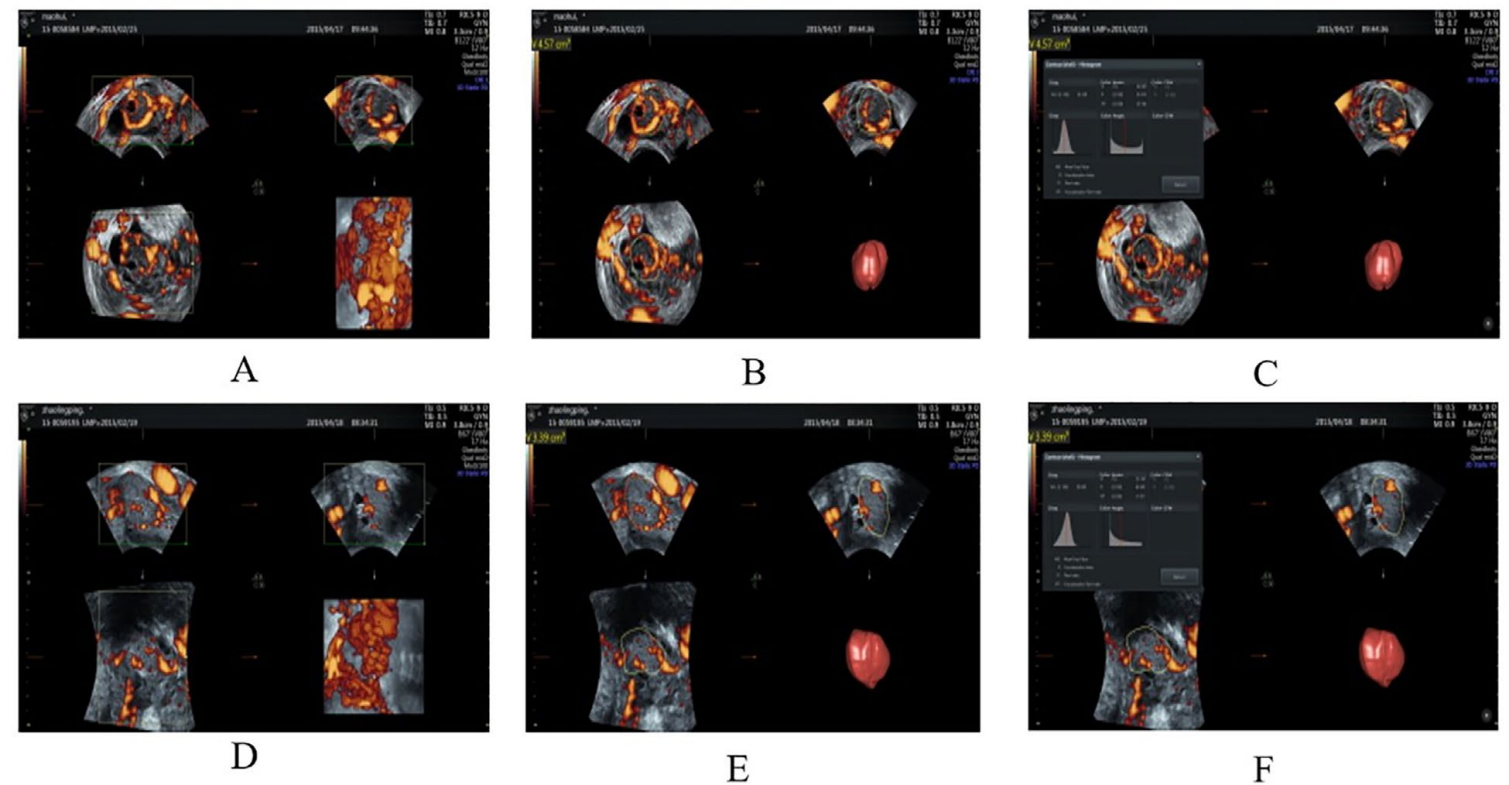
Three-dimensional ultrasound images of corpus luteum in group A and group B. (A, B) The 3D-PD ultrasound images of the corpus luteum in group A (27 y, G1P0, menolipsis 69 days, no symptoms of abdominal pain or vaginal bleeding, intrauterine pregnancy, having yolk sac, germ, and fetal heart beating). (C) The histogram of gestational corpus luteum in the same patient. (D, E) The 3D-PD ultrasound images of the corpus luteum in group B (34 y, G1P0, menolipsis 59 days, with the symptoms of abdominal pain and vaginal bleeding, intrauterine pregnancy, having yolk sac and germ, no fetal heart beating). (F) The histogram of gestational corpus luteum in the same patient. 3D-PD indicates the three-dimensional power Doppler.
